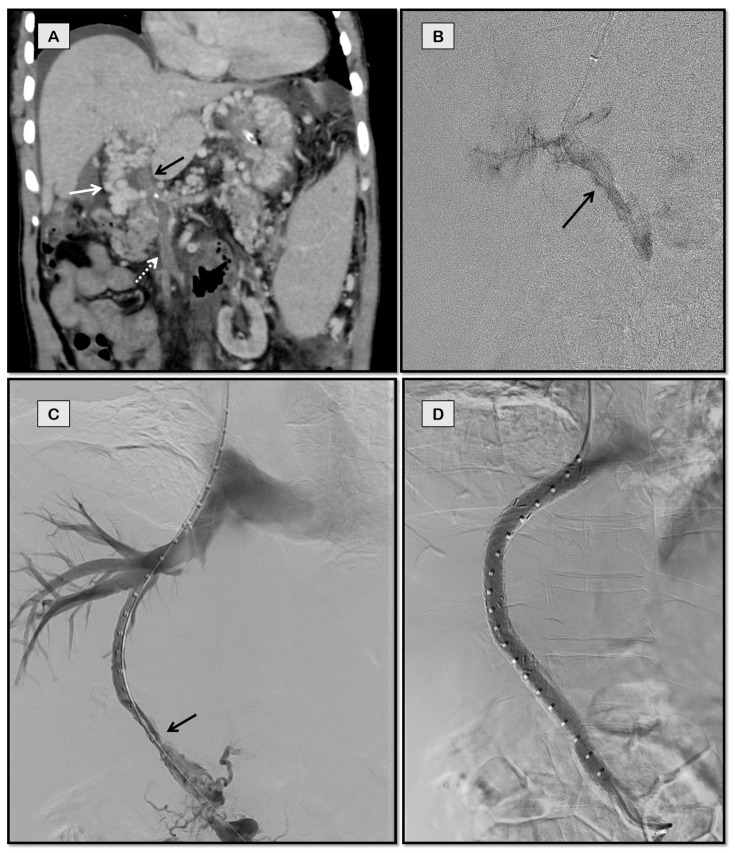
TIPS in a 61-year-old man with polycythemia vera and chronic porto-mesenteric vein thrombosis who presented with recurrent variceal bleed refractory to endoscopic therapy. Patient was not a surgical candidate due to multiple comorbidities. Coronal-oblique image (A) from the computed tomographic (CT) scan of the patient showing chronic thrombosis of the portal vein (black arrow) and superior mesenteric vein (dashed arrow) with cavernoma formation (white arrow). Fluoroscopic spot image (B) shows the thrombosed portal vein (arrow) accessed from jugular approach. Post balloon maceration and thromboaspiration, there is partial recanalization of the portal and superior mesenteric vein (C). Post-stenting (D) image shows brisk flow through the stent with absence of collateralization.
